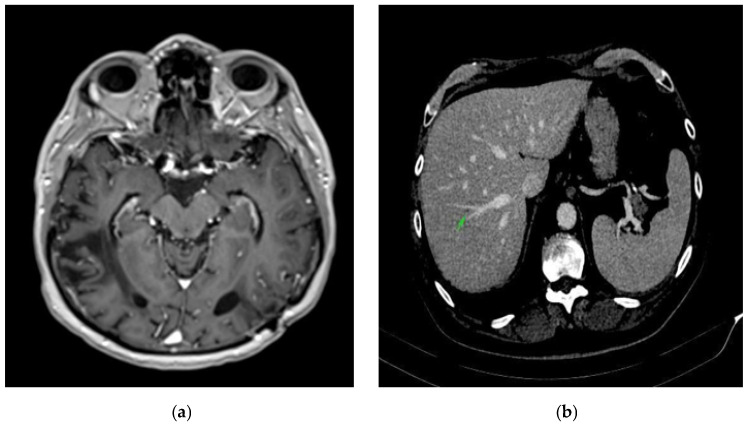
Complete remission. (a) Brain CT scan: complete remission of occipital lobe metastases. (b) Abdominal CT scan: complete remission of hepatic metastases.
